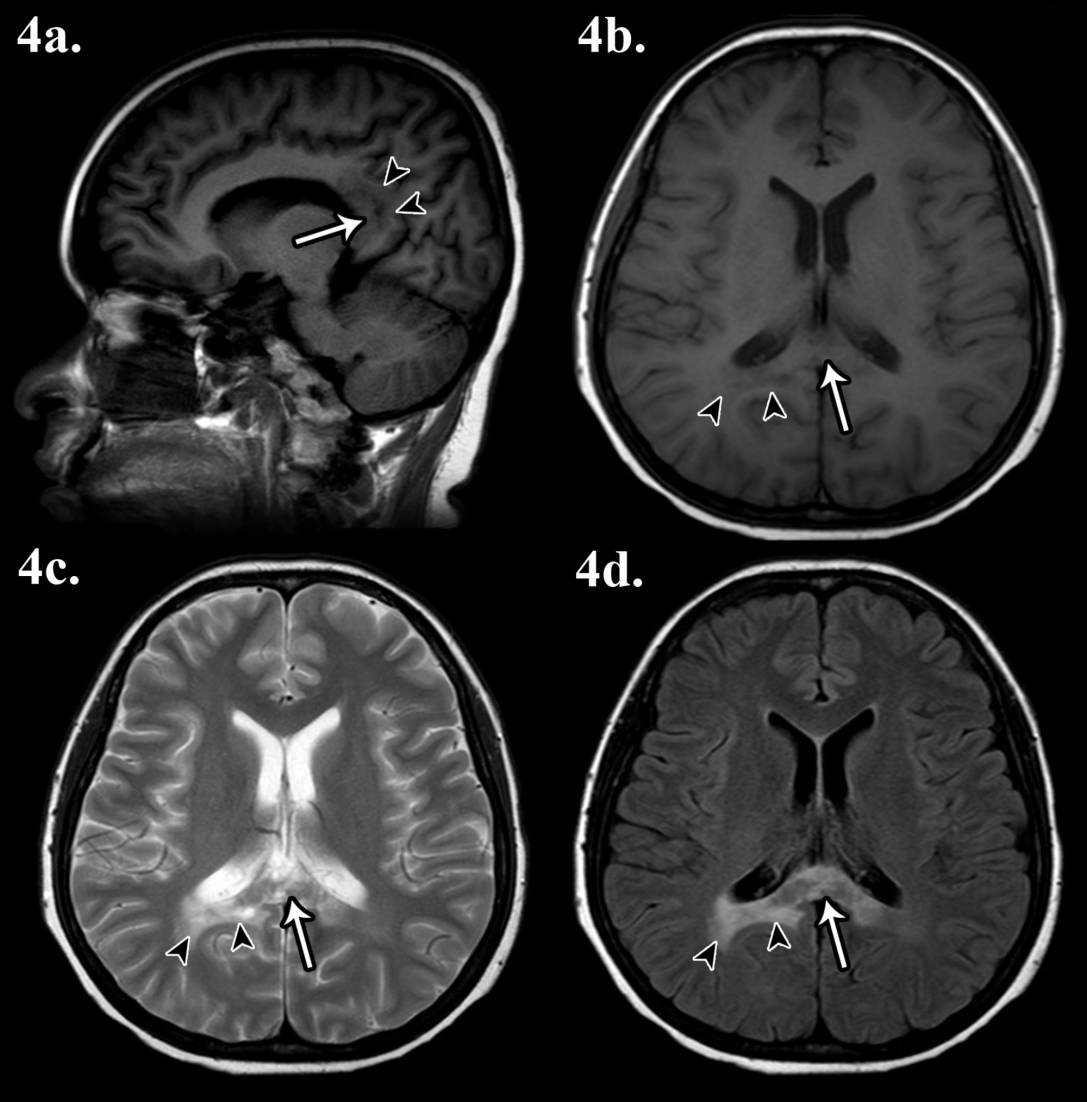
Magnetic resonance imaging (MRI) of the brain post chemotherapy 4a) T1-weighted sagittal imaging; 4b) T1-weighted axial imaging; 4c) T2-weighted axial imaging; 4d) fluid attenuation inversion recovery (FLAIR) sequence imaging. Marked reduction in the size of the previously seen mass at the splenium of corpus callosum (white arrow), ​​​​​​with reduced white matter oedema (black arrowheads).
